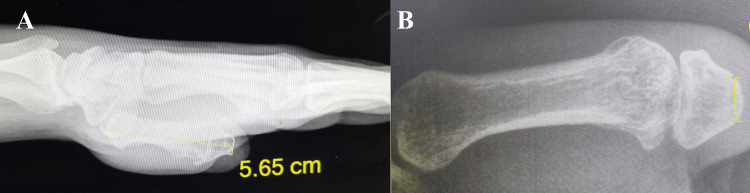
Radiographic images of the patient before the treatment (A, B) Radiographic images showing amputation at the base of the proximal phalanx of the thumb.
